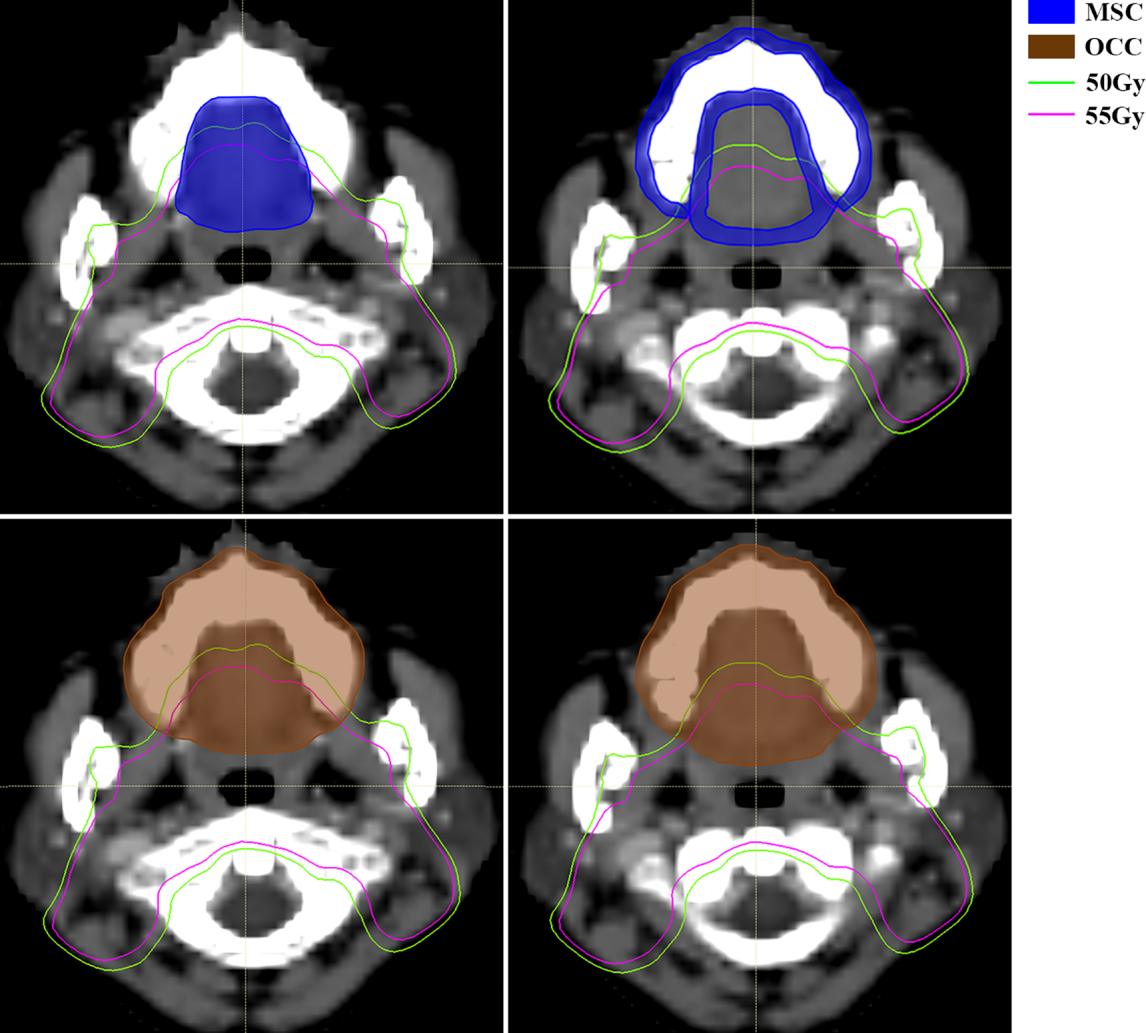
Computed tomography (CT) scan of a nasopharyngeal carcinoma patient with mucosal surface contours (MSC) (up, area filled with blue) and oral cavity contours (OCC) (down, area filled with brown). MSC involves the mucosal surface while OCC encompass more solid tissue, like tongue, maxillary bone, etc. The green line and pink line are isodose curve of 50Gy and 55Gy respectively. Abbreviations: OCC, oral cavity contouring; MSC, mucosa surface contouring.
